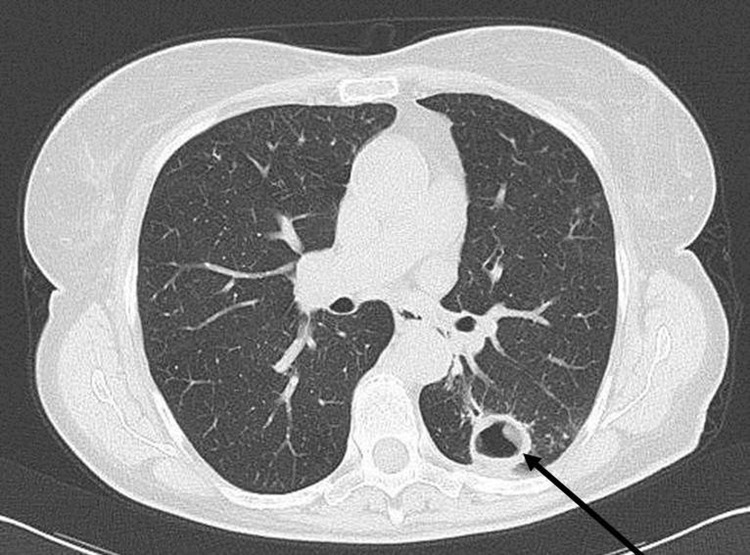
Computed tomography scan showing a cavity (arrow) in the left lung of a 74-year-old woman (patient 1) in Italy. Laboratory testing suggests that the woman was infected with *Mycobacterium yongonense*.
